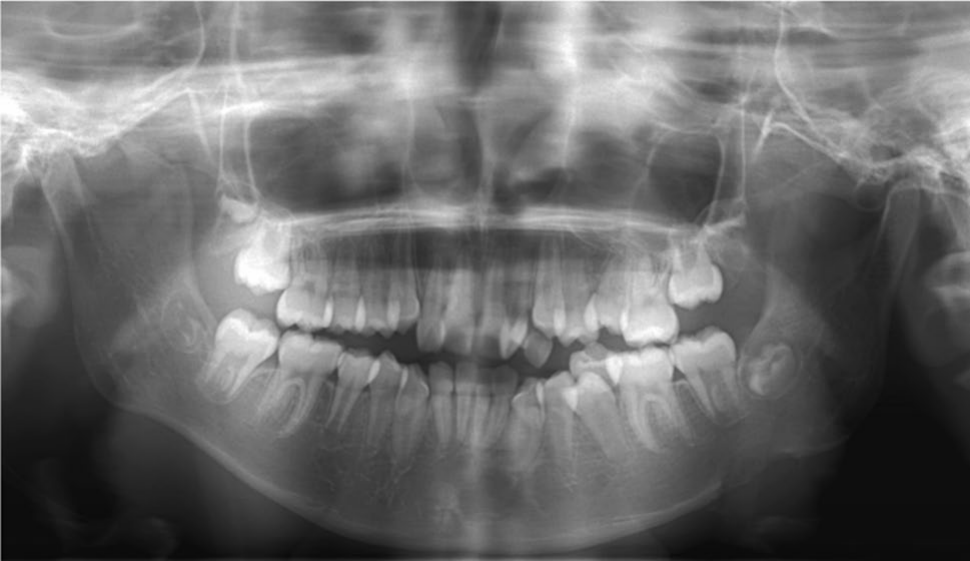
Panoramic radiography of the lesion in the left mandible. Panoramic radiography showing a rounded radiolucent lesion with irregular border
